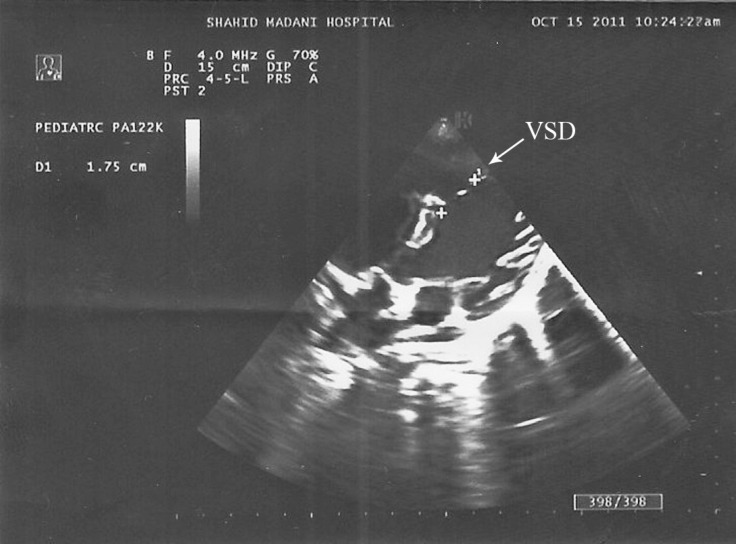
Parasternal short-axis view of the heart by epicardial echocardiography shows the large apicomuscular VSD.
